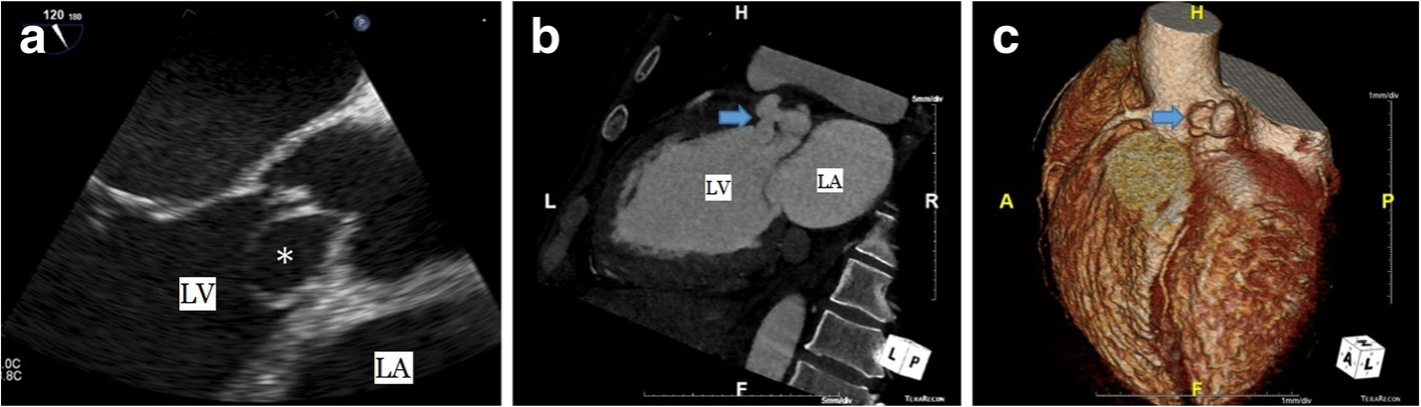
a Transthoracic echocardiography shows the sac in the left ventricle (asterisk). b, c Multidetector computed tomography shows a ruptured pseudoaneurysm of sinus of Valsalva originated from left coronary sinus into left ventricle (arrow). LV, left ventricle; LA, left atrium
